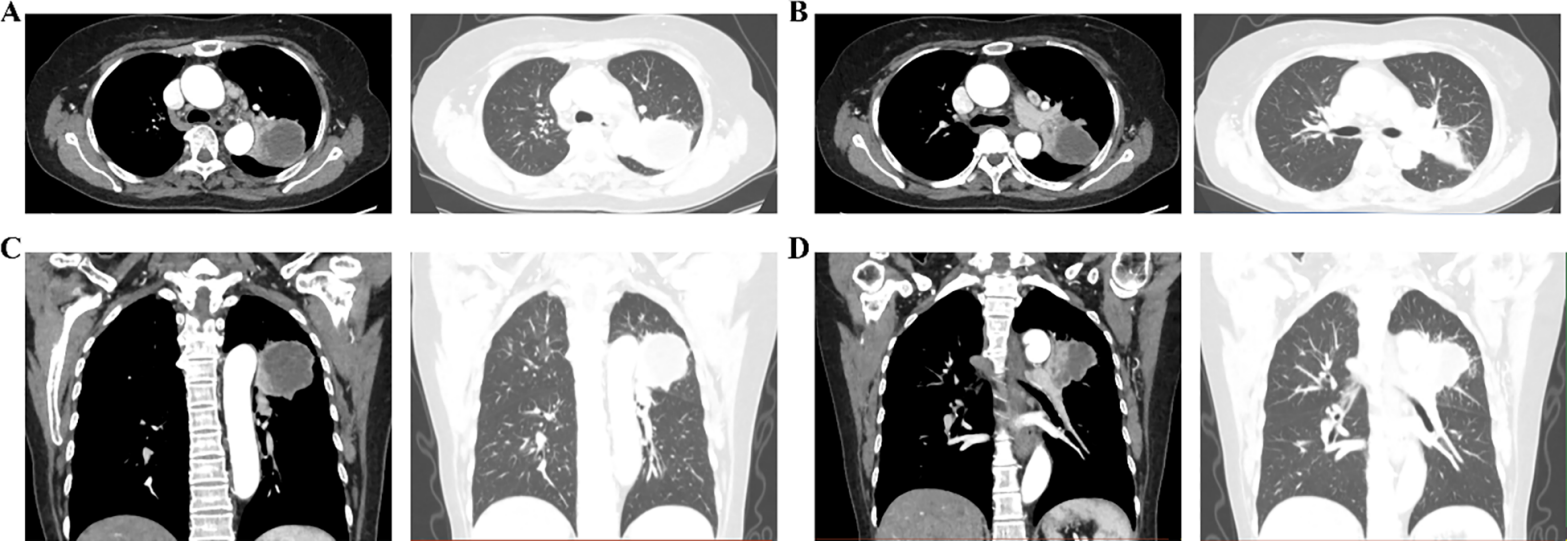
Enhanced chest CT showing the upper lobe lesion in the left lung. (A, B) Mediastinal and lung windows at different levels; (C, D) Three-dimensional reconstruction of lesion distribution.
